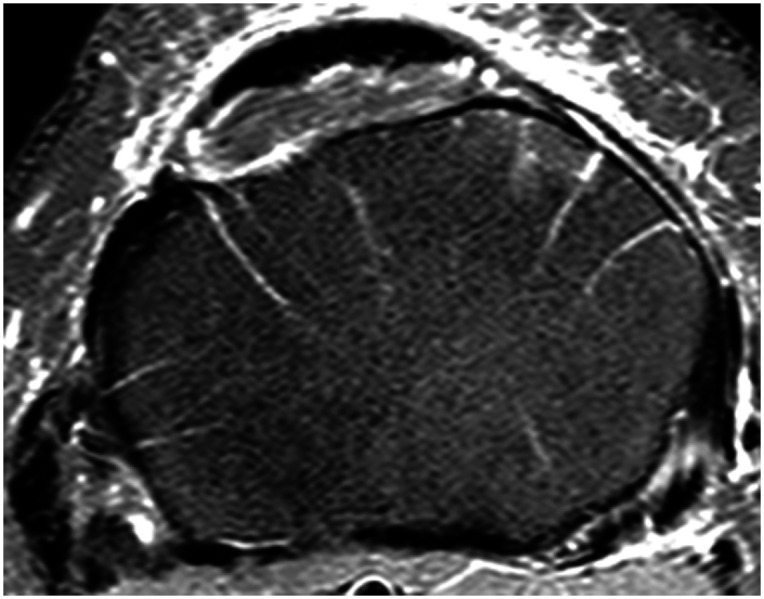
Normal proximal tibial slice from a PD_SPAIR MRI scan showing the radiating water bright vascular marks.
